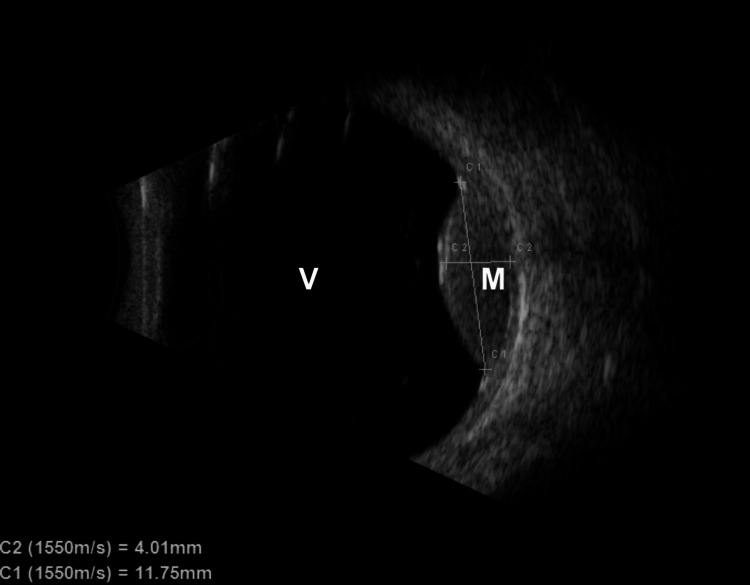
Ultrasound eye examination before treatment. V: vitreous, M: melanoma.
